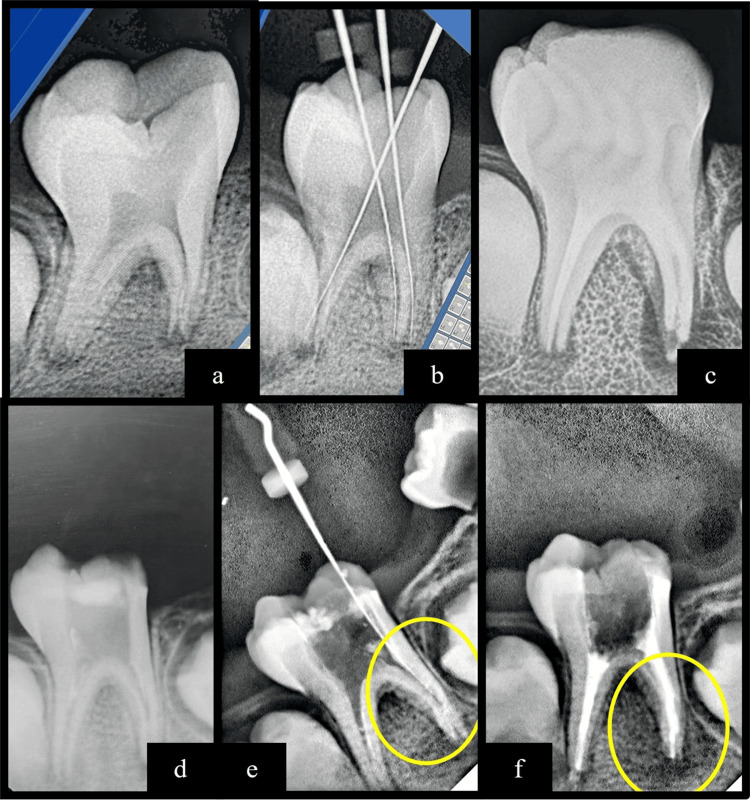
(a) Preoperative radiograph with respect to tooth #46. (b) Working length determination. (c) Metapex medicament for apexification (duration: six months). (d) Metapex removal. (e) Working length determination showing calcification after Metapex removal. (f) Completion of root canal therapy.
